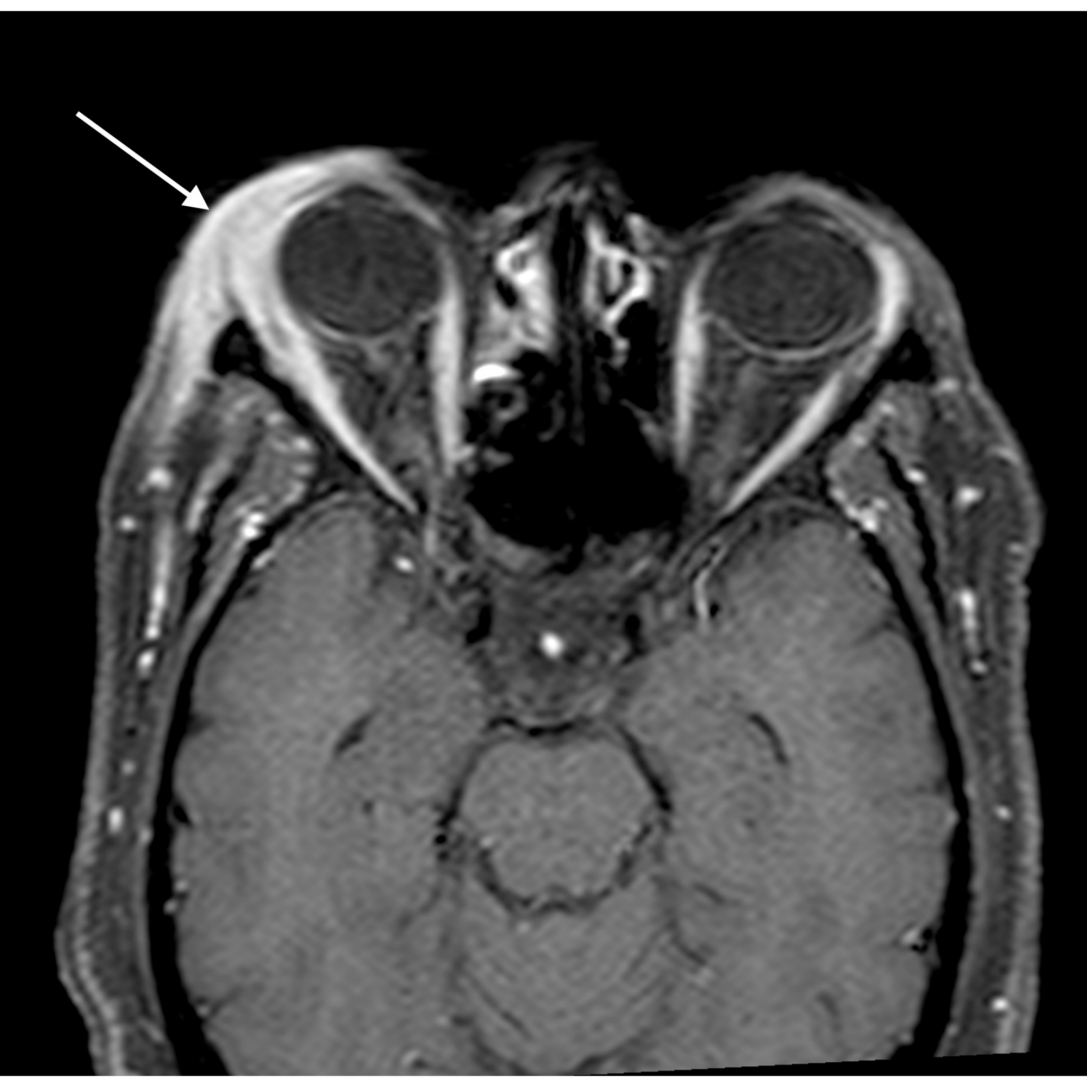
Periorbital cellulitis: axial post gadolinium T1WI shows abnormal enhancement of the preseptal soft tissue thickening (arrow) and extending in the infratemporal fossa T1WI - T1-weighted image
